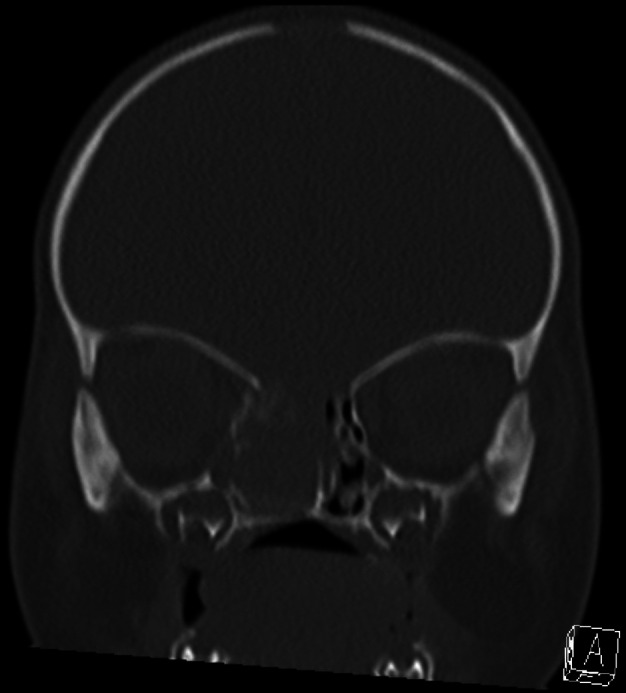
Invasion of the bony border of the orbit and scarification of the frontal skull base in CT scan in coronal plane
